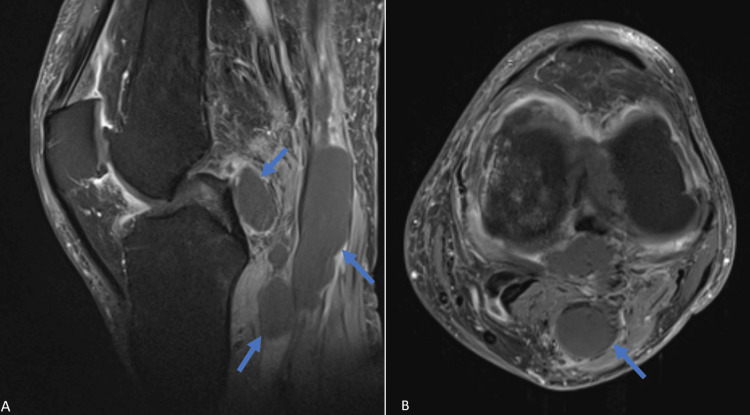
Preoperative T1-weighted contrast-enhanced MRI of the left knee A: Preoperative T1-weighted contrast-enhanced MRI of the left knee, sagittal scan, showing the cystic lesions (blue arrows) with no pathologic contrast-enhancement. B: Preoperative T1-weighted contrast-enhanced MRI of the left knee, axial scan, showing a well-circumscribed cystic lesion (blue arrow) with no pathologic contrast-enhancement.
